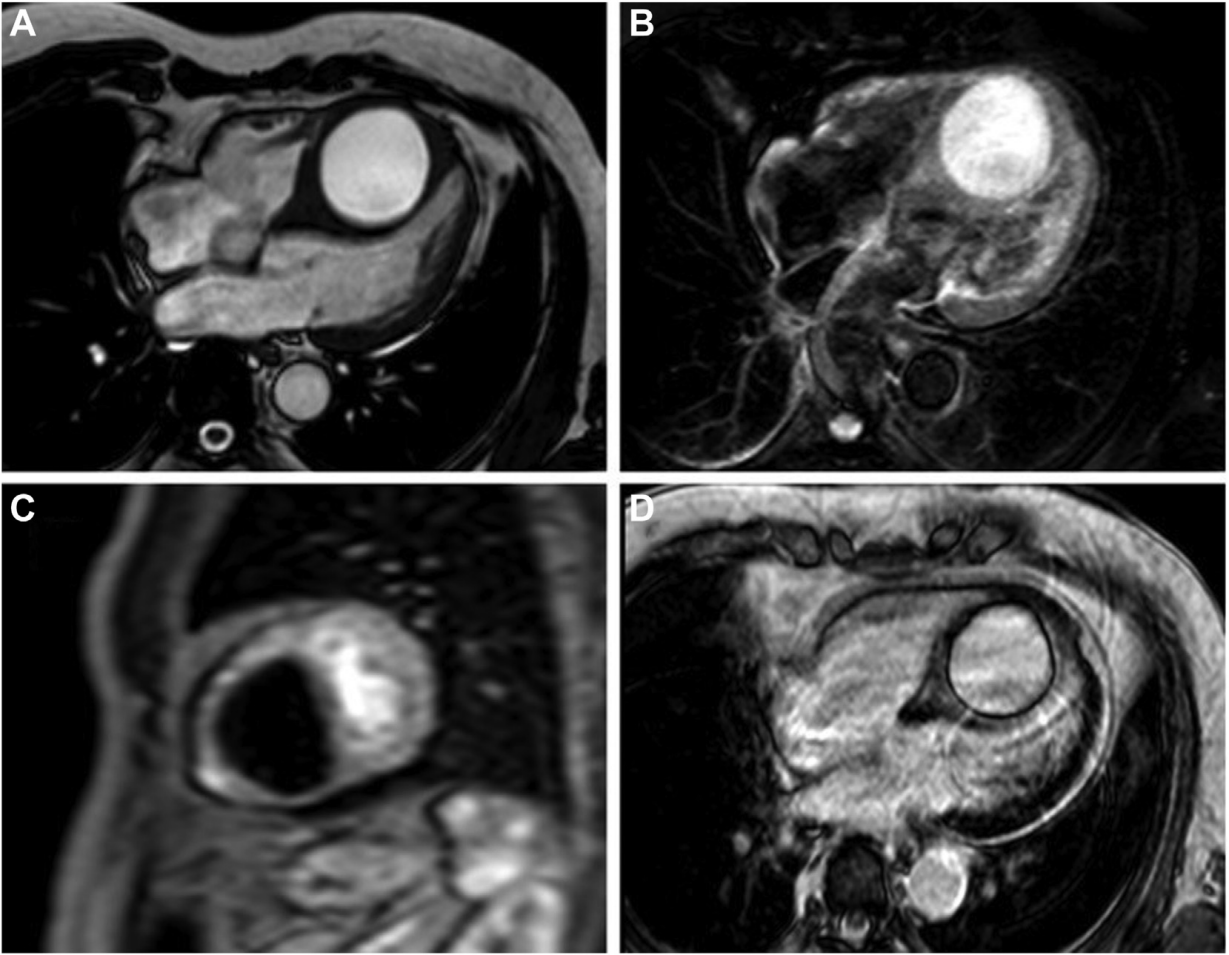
Cardiovascular magnetic resonance images demonstrating a large cyst in the IVS with (A) hyperintensity on T1-weighted acquisition, (B) hyperintensity on T2-weighted acquisition, (C) absent perfusion during intravenous gadolinium administration (short-axis images), and (D) no delayed gadolinium enhancement on the thin cystic wall.
